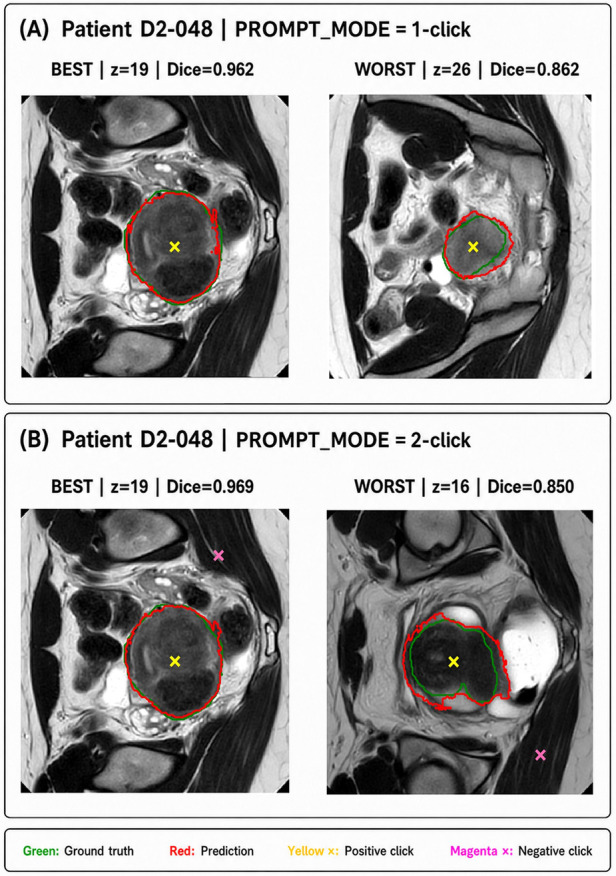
Single-patient qualitative example of uterus segmentation by Endo-MedSAM on pelvic MRI for Patient D2-048 from the D2 cohort. The figure is divided into two panels showing interactive click-prompting modes: (A) 1-click and (B) 2-click. Each panel presents the best-performing slice on the left and the worst-performing slice on the right, with the slice index (z) and Dice score displayed above each image. Green contours denote the ground-truth uterus mask, and red contours denote the Endo-MedSAM predicted segmentation. Colored cross markers indicate the interactive click prompts used for segmentation.
